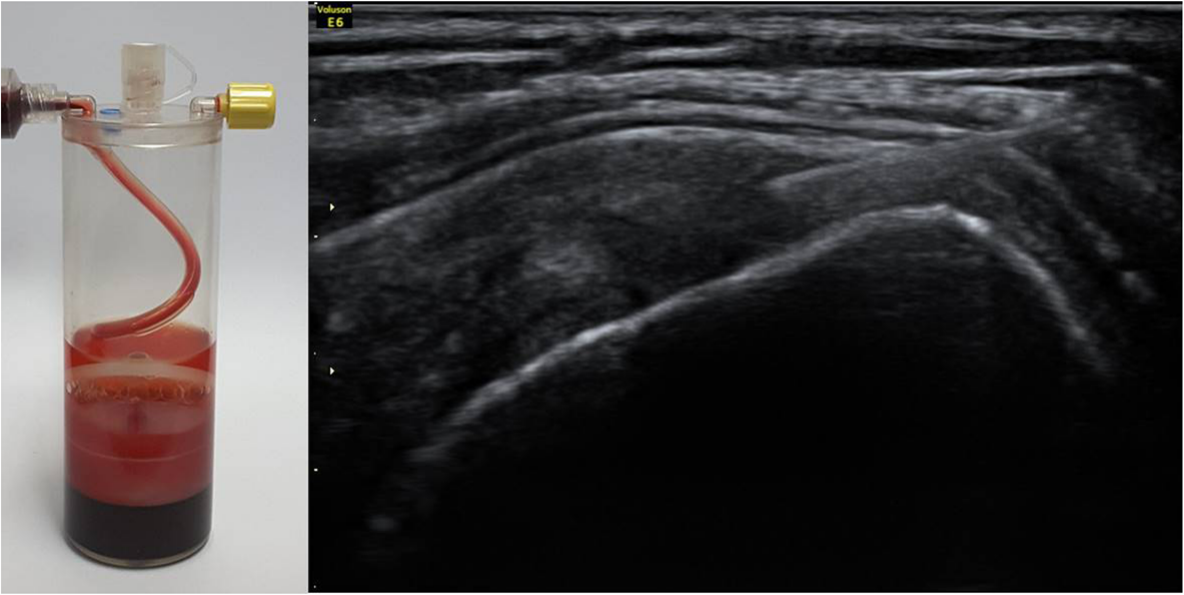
Bone marrow aspirate concentrates (BMAC) were acquired from the bone marrow and centrifuged with a BIOMET MarrowStim™ Mini kit to isolate the concentrated BMACs (left side). The patients received the BMAC-PRP injection at the tear site under ultrasound guidance by one experienced physiatrist (right side)
